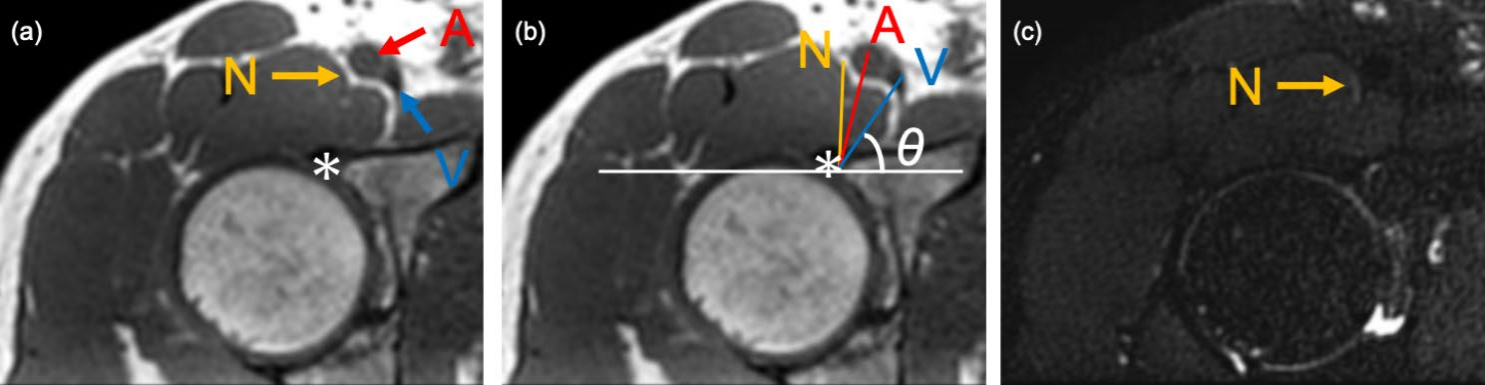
Magnetic resonance (MR) image (a) showing the femoral artery (A), femoral vein (V), and femoral nerve (N) as well as the anterior commissure (*). MR image showing the distance and angle measurements of the femoral neurovascular bundle from the acetabulum (b). Nerve-enhanced image showing the location of (N) (c)
